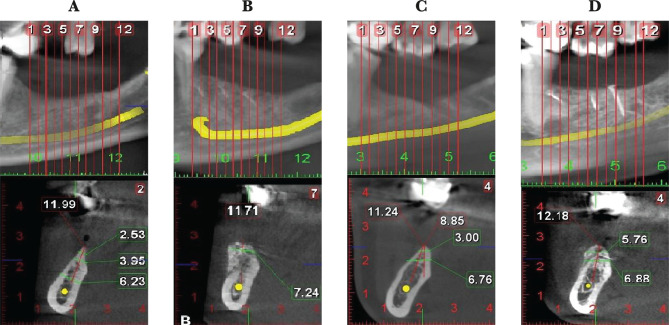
CBCT sections of the initial (A, C) and the augmented surgical site, 6 months after the surgery, with sticky bone (B) and bone block (D).
